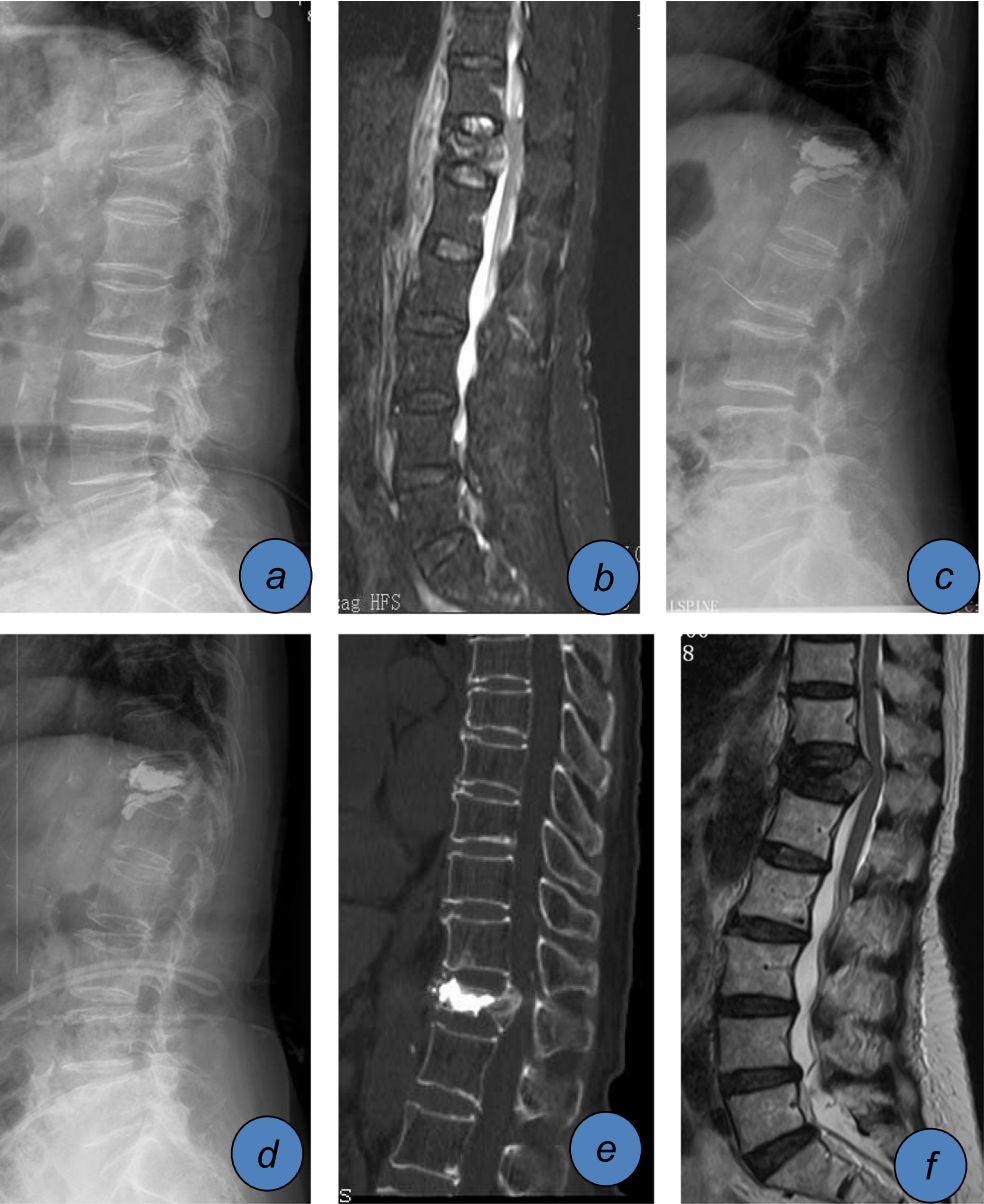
An 80-year-old female patient with thoracic 12 vertebral compression fractures underwent PKP. BMD T = − 3.3. a X-ray showed a wedge-shaped change of T12. b MR showed a fresh compression fracture of T12, with IVC. c After PKP surgery, the X-ray showed that there was no obvious collapse of the T12 vertebral body. d After 3 months, the X-ray showed that the T12 vertebral body had local collapse and kyphosis aggravation. e The sagittal plane CT showed that the T12 vertebral body collapsed and the posterior wall of the vertebral body squeezed the spinal canal. f MR showed that the spinal canal and spinal cord were compressed
